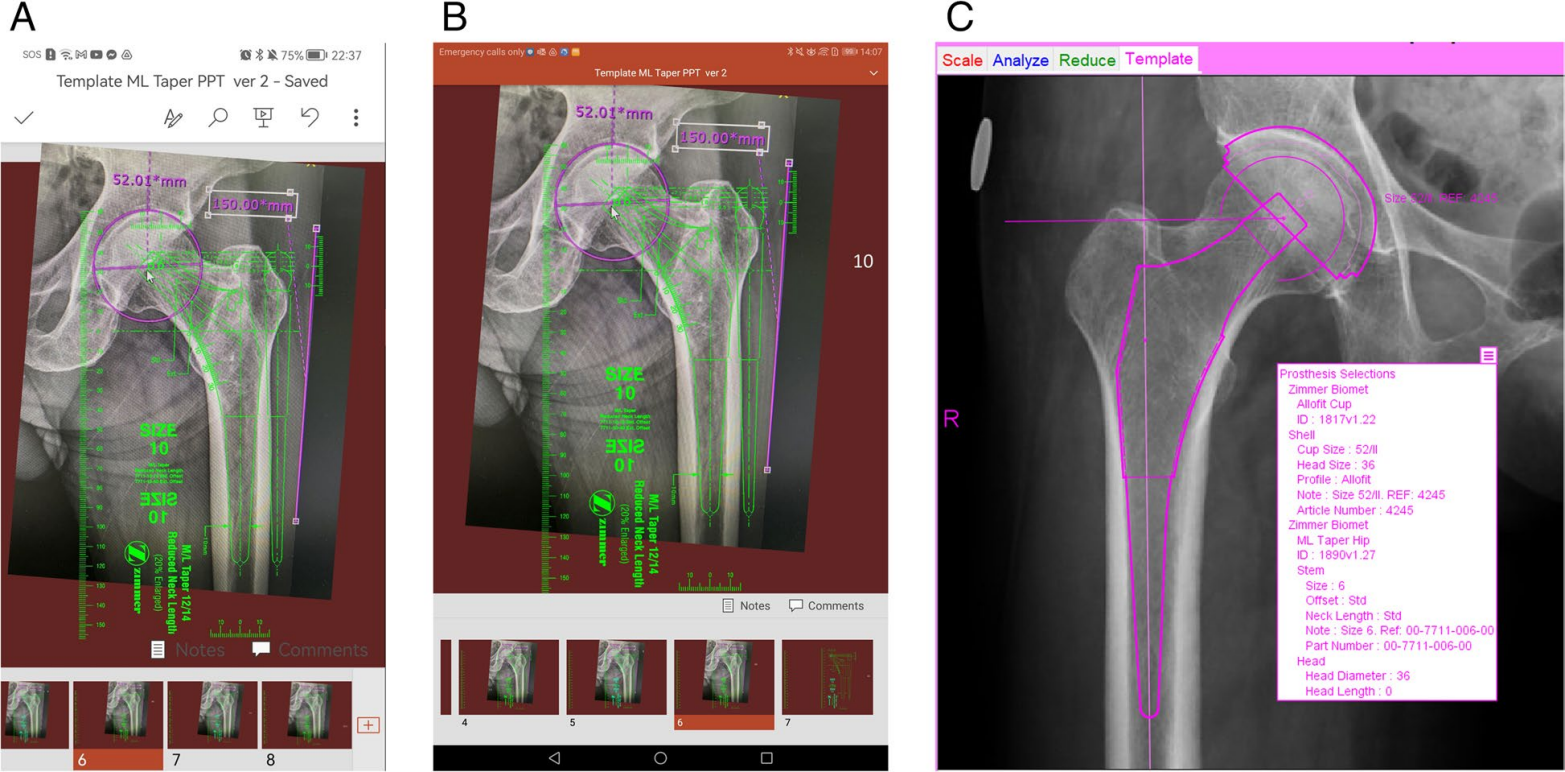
A Femoral stem templating using the PowerPoint 365® mobile application on a smartphone. B Femoral stem templating using the PowerPoint 365® application on a tablet. C Hip radiograph with overlaid digital templates and a summary table of results from OrthoView® software
